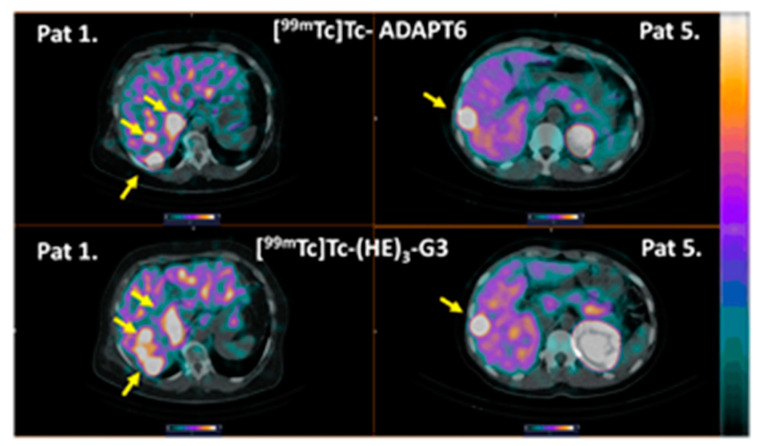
Imaging of liver metastases in HER2-positive breast cancer patients 1 and 5. Arrows point at metastases. The upper setting of a linear intensity scale is adjusted to SUV 6.8 in all images.
